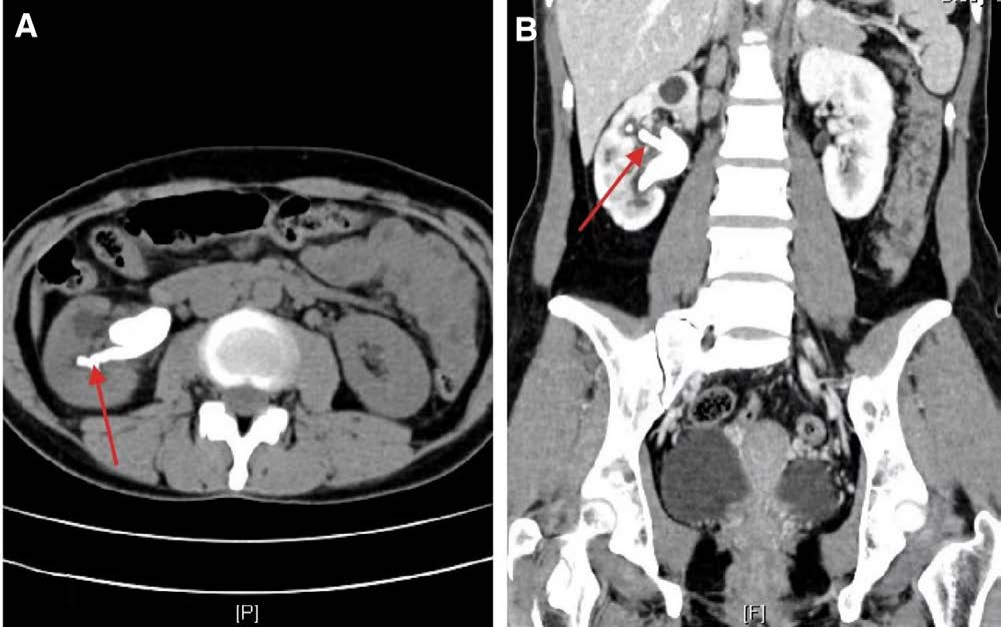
The red arrows in the transverse (A) and coronal (B) planes of CT show elongated branches of staghorn calculi. CT = computed tomography.
